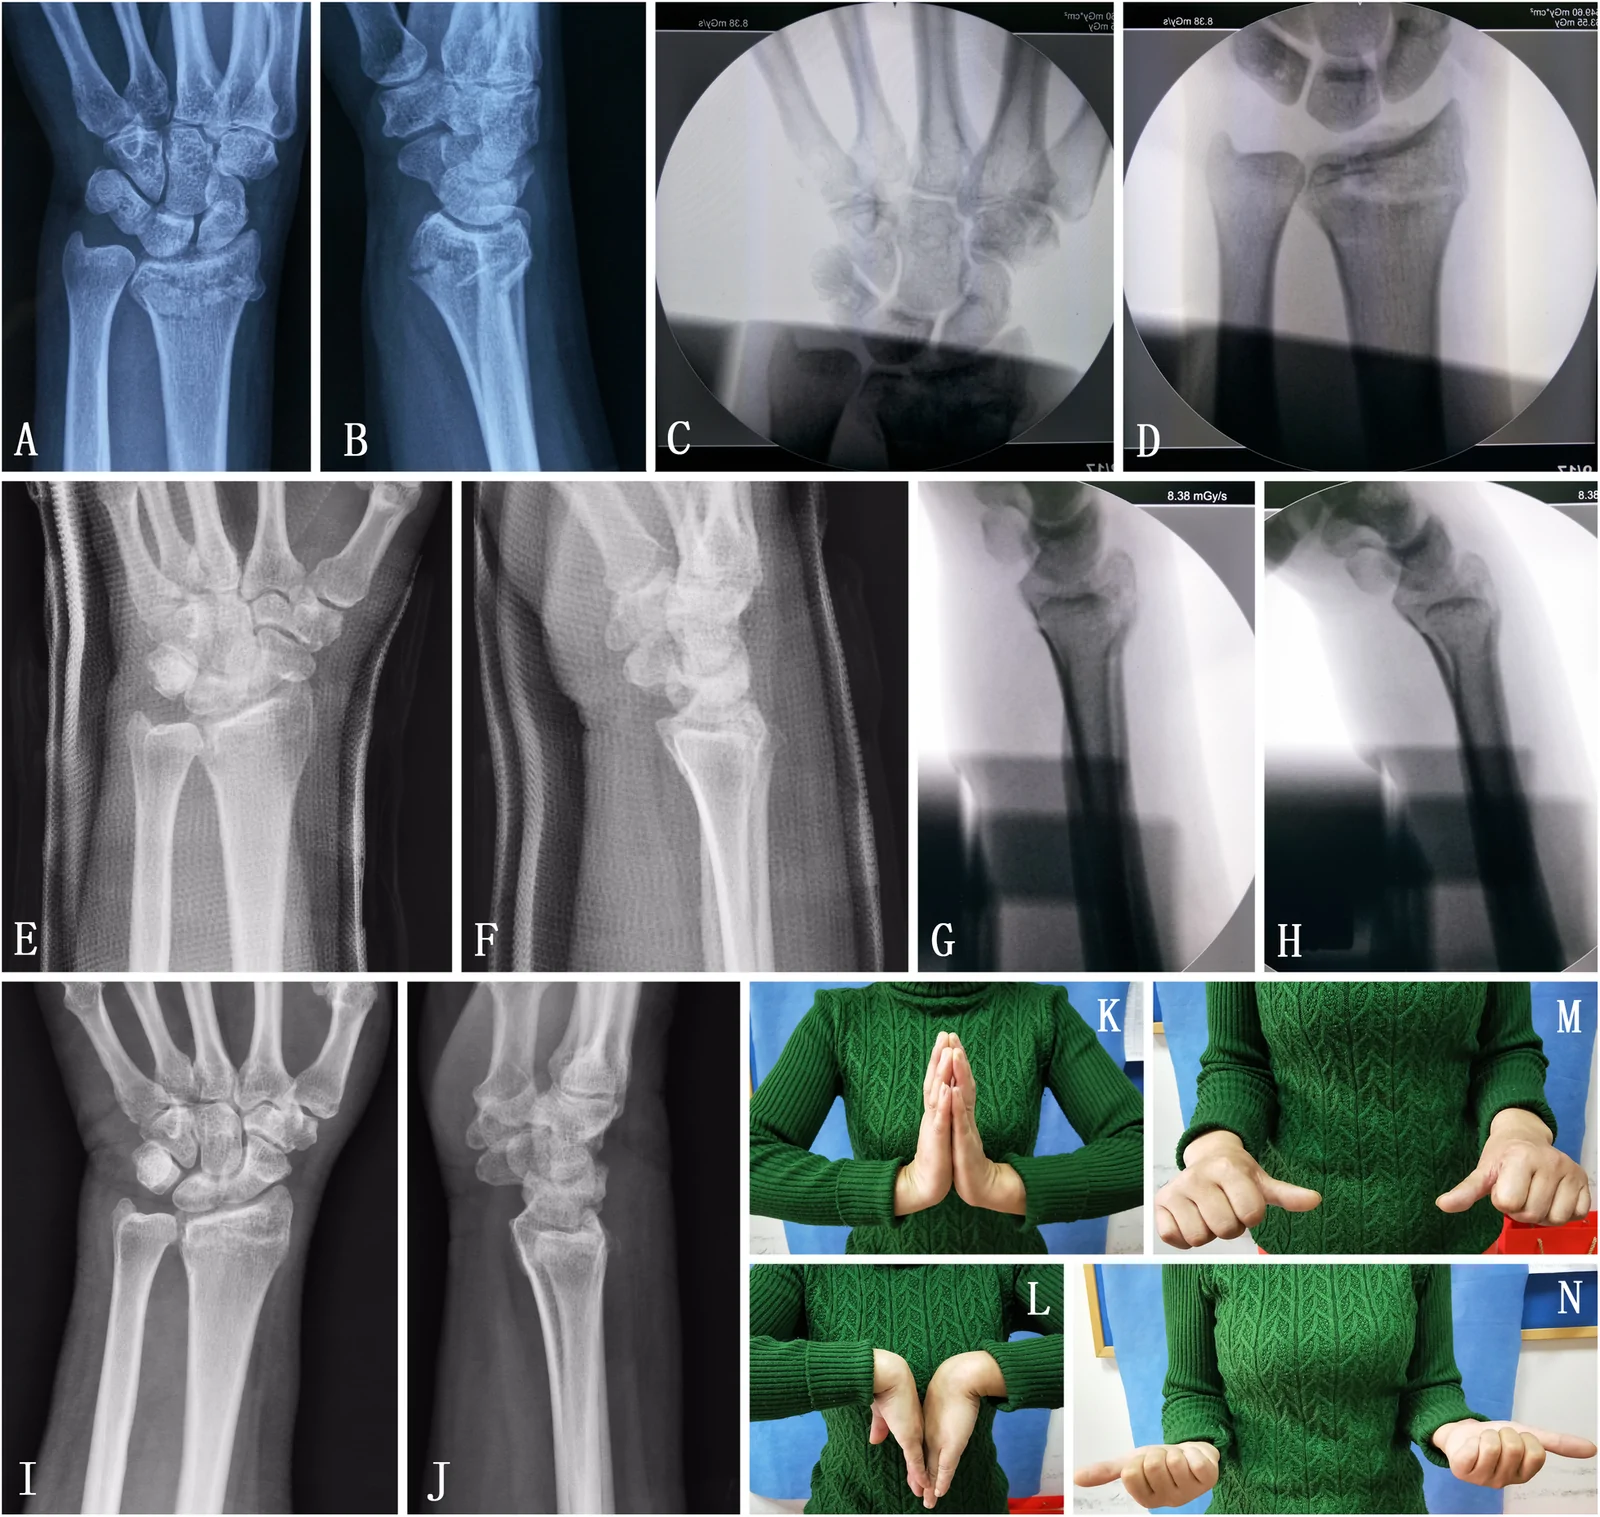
A 70-year old female fell down from a standing height and suffered from fracture of left distal radius. (A,B) x-ray views of the fracture. (C,D) The anteroposterior fluoroscopy image of fracture site when the distal fragment was gradually reduced by traction and ulnar deviation by operating the device. (G,H) The fluoroscopy image of fracture site when the distal fragment was gradually reduced by flexion by operating the device. (E,F) The immediate x-ray of fracture after reduction and cast immobilization. (I,J) The x-ray of fracture at 6-month follow up. (K–N) The ROM of injured wrist at 6-month follow-up.
